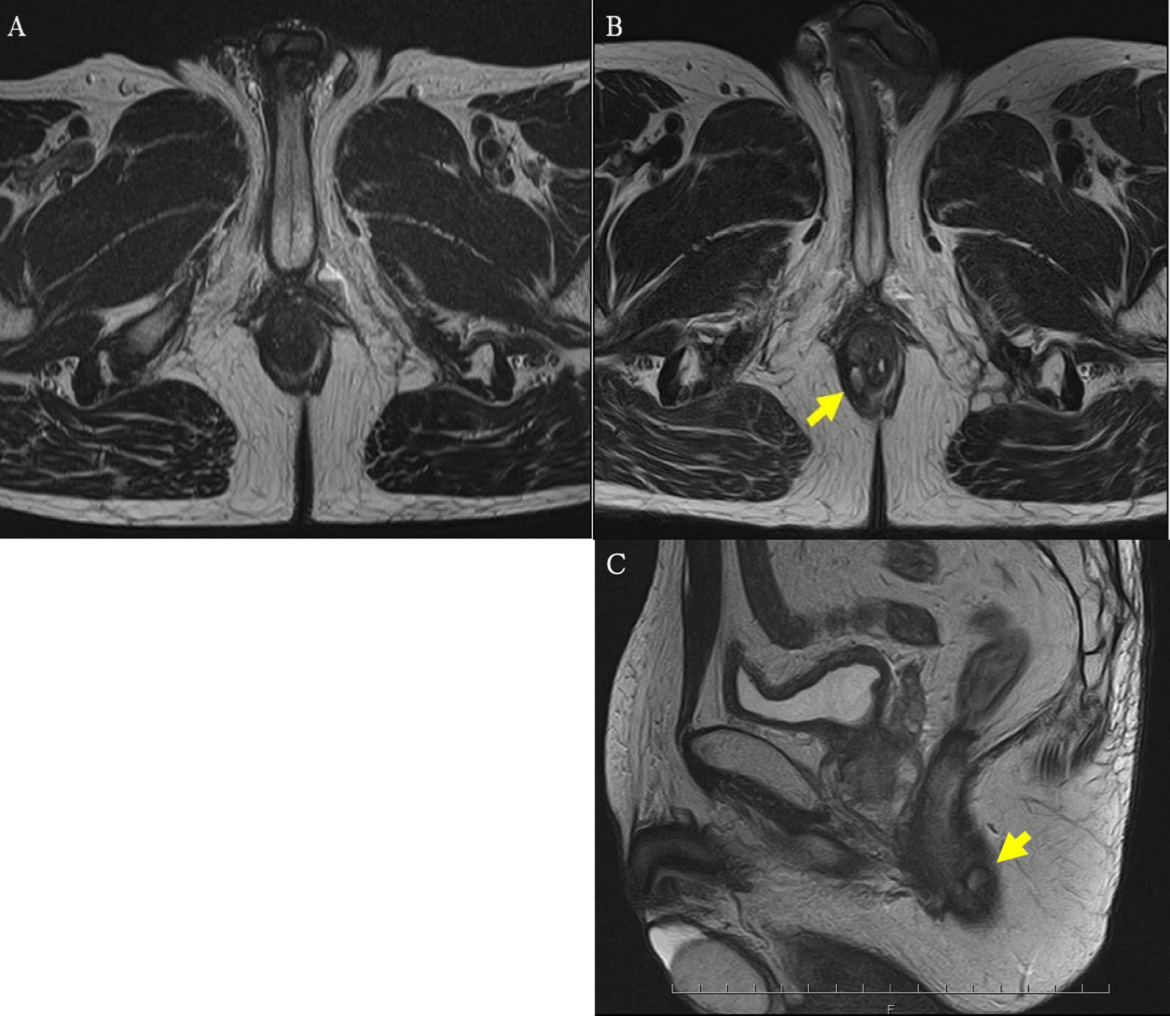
T2-weighted magnetic resonance imaging of the anal fistula. A Image obtained before preoperative chemoradiation therapy. No anal fistulas are observed. B, C Image shows a high-intensity anal fistula after chemoradiation therapy, located from the anal crypt to the skin behind the anus (arrows)
